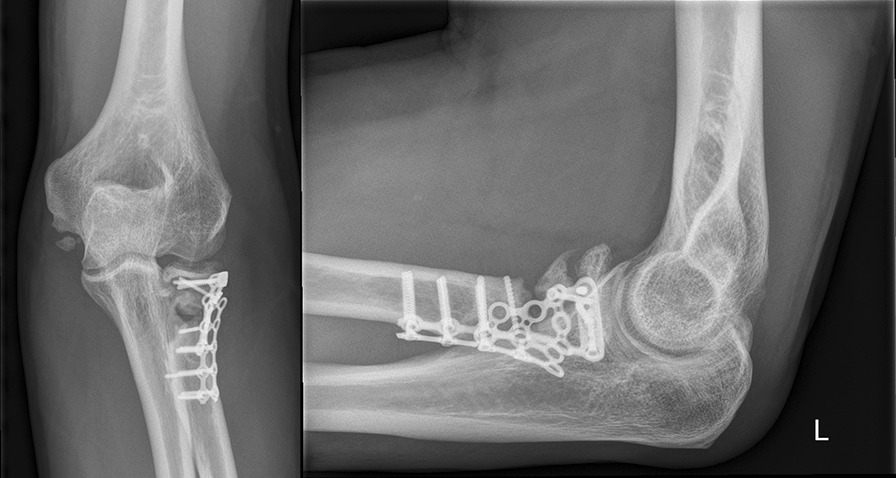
Final radiographs (sagittal range of motion: 0–5–130°; rotational range of motion 65–0–45°; MEPI 85; DASH 16)
